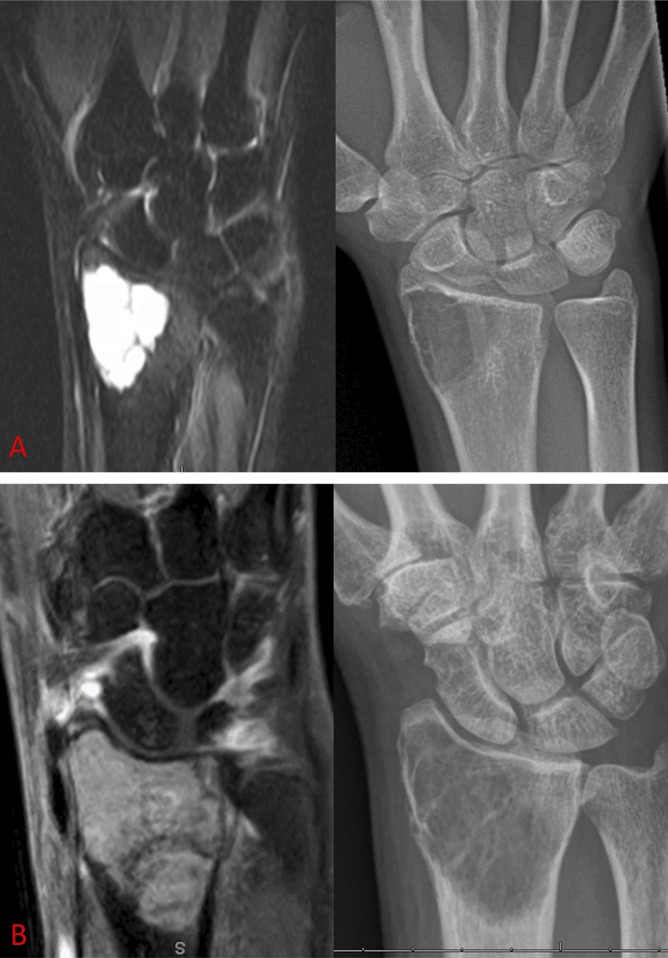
Examples are shown of paired MRIs and radiographs of GCTs of bone, which were downgraded in both raters from (A) Campanacci grade 2 to novel grade 1 and (B) Campanacci grade 3 to novel grade 1.
